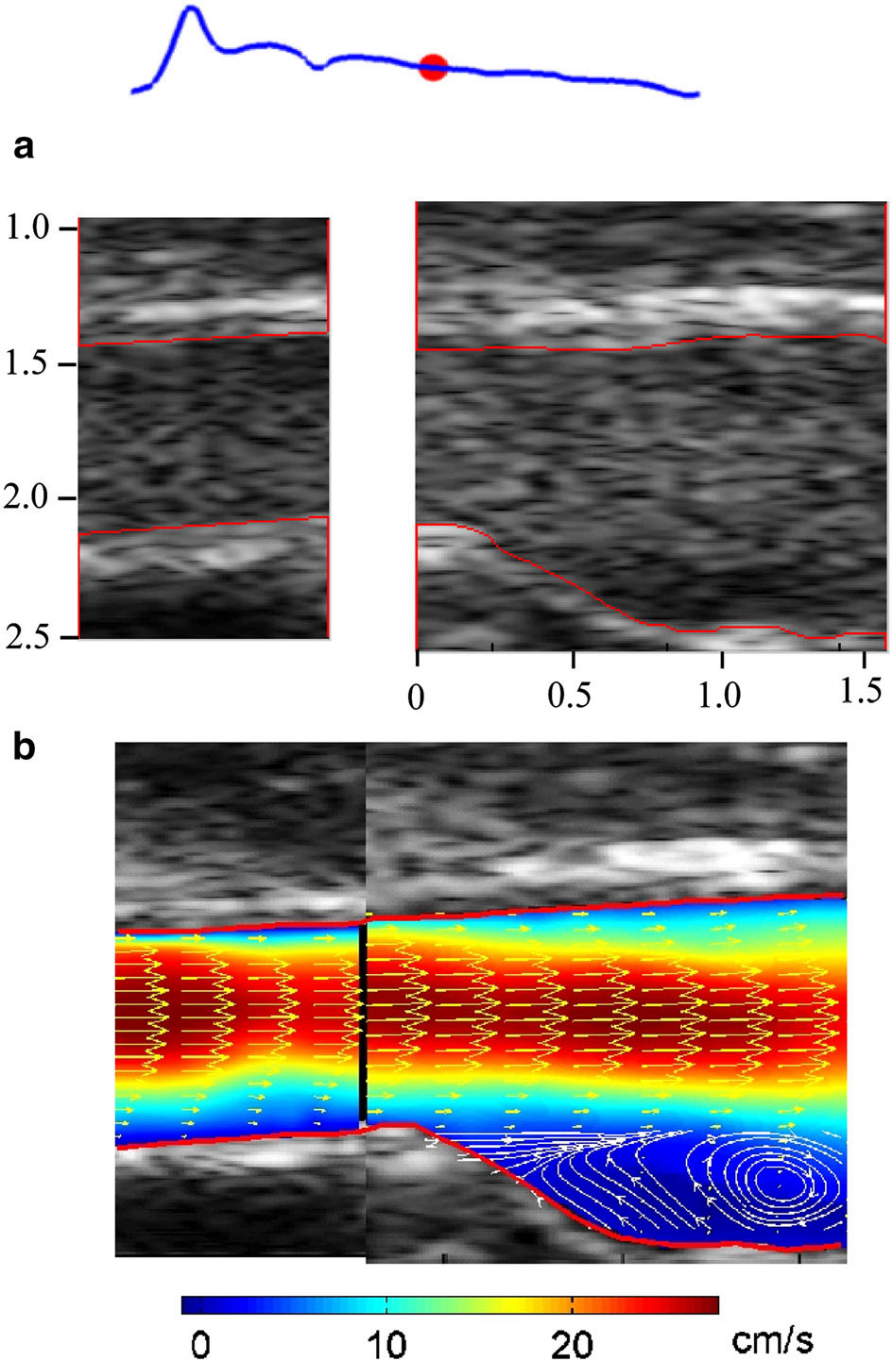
In vivo measurement of the velocity in a carotid artery using ultrasound imaging velocimetry/echo-PIV a raw ultrasound images (using contrast medium). The tick marks have units of centimetres, b instantaneous velocity field, combining data from two measurements. The colours represent the velocity magnitude. The red marker at the top indicates the time instance during the cardiac cycle (blue curve). Reproduced from Zhang et al. [86] with permission ©2011 Elsevier (color figure online)
